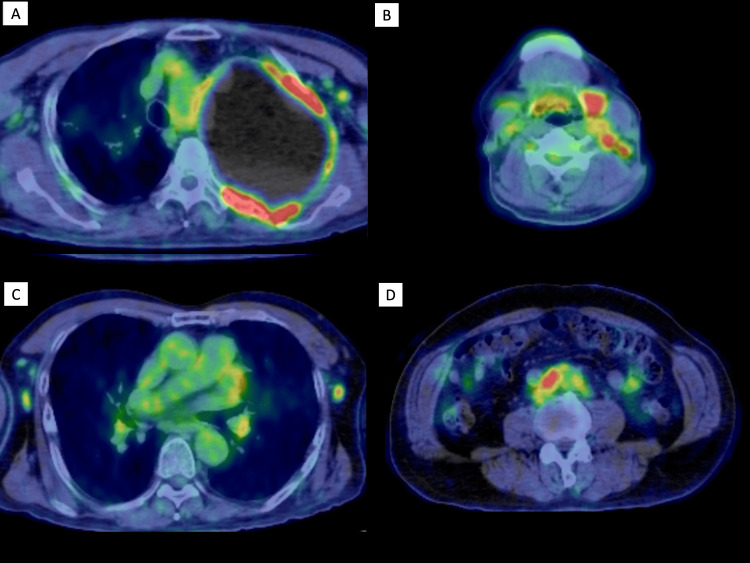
FDG PET-CT at admission. ^18^F-FDG PET-CT revealed the abnormal accumulation of FDG in a soft tissue mass around the plombage (A), salivary glands and cervical lymph nodes (B), axillary lymph nodes (C), and a retroperitoneal soft tissue mass around the abdominal aorta (D). Abbreviation: ^18^F-FDG PET-CT, ^18^F-fluorodeoxyglucose positron emission tomography-computed tomography.
